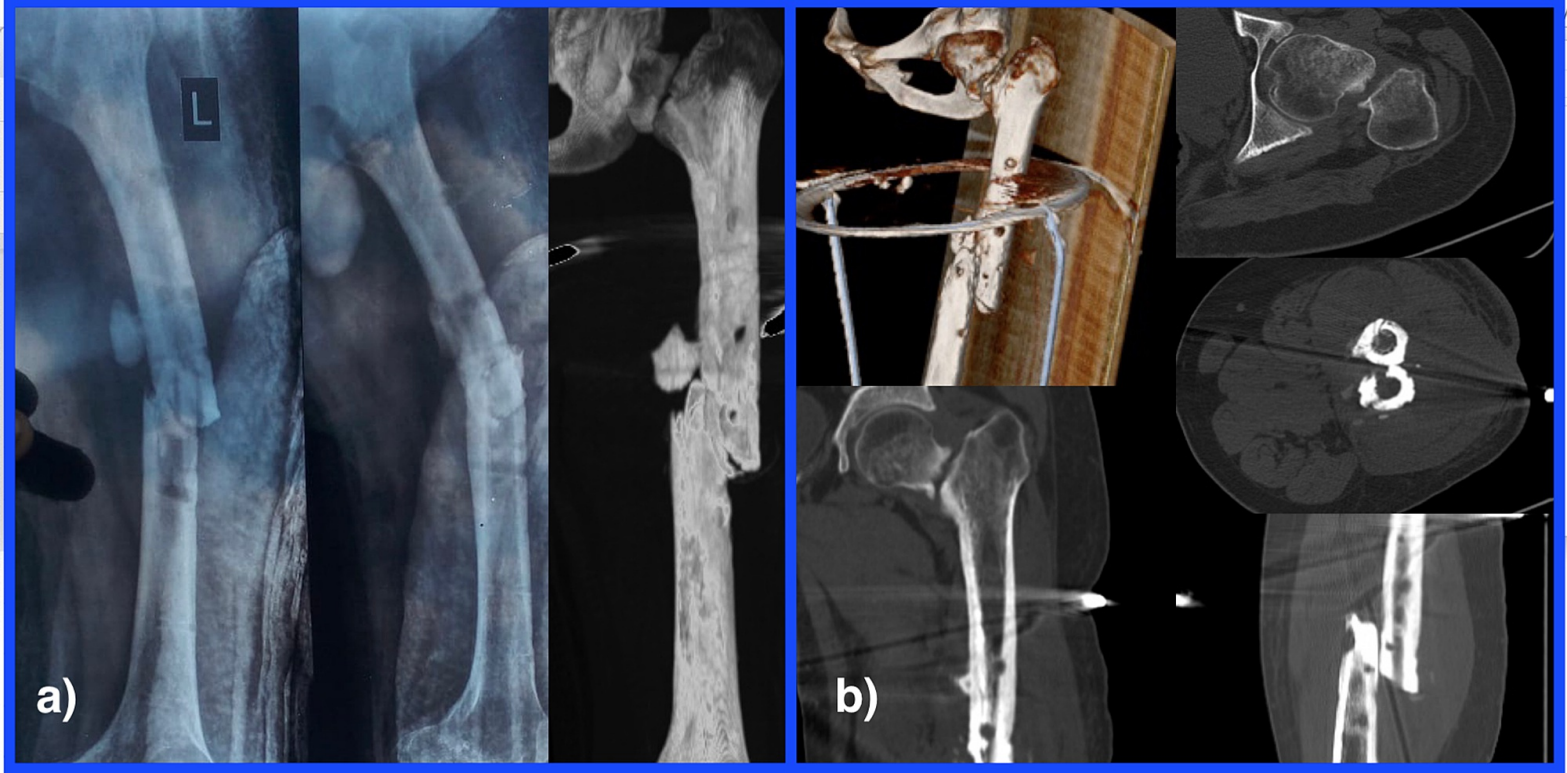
Non-union of the ipsilateral neck of femur and shaft of femur left side. a) Preoperative radiographs reveal non-union of shaft femur fracture without any evidence of healing. The neck of the femur radiograph reveals sclerotic and smooth margins along with the gap at the fracture site. b) the computed tomography 2D and 3D images confirm the findings of atrophic non-union of the shaft of femur and neck of femur.
